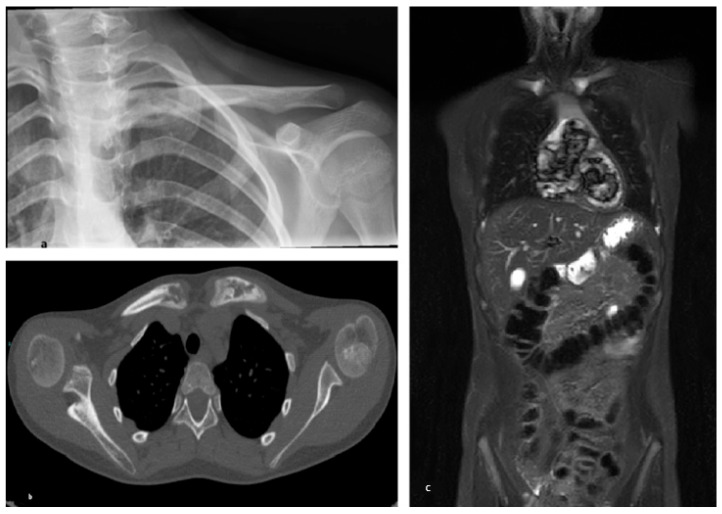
Patient 1, diagnosed with JIA in overlap with CNO. (a) X-ray of the left clavicle showing focal osteolytic changes at the medial end of the left clavicle; (b) CT scan showing osteolytic changes at the sternal ends of both clavicles; (c) Whole-body MRI (STIR) showing focal hyperintensity at both medial clavicular ends.
